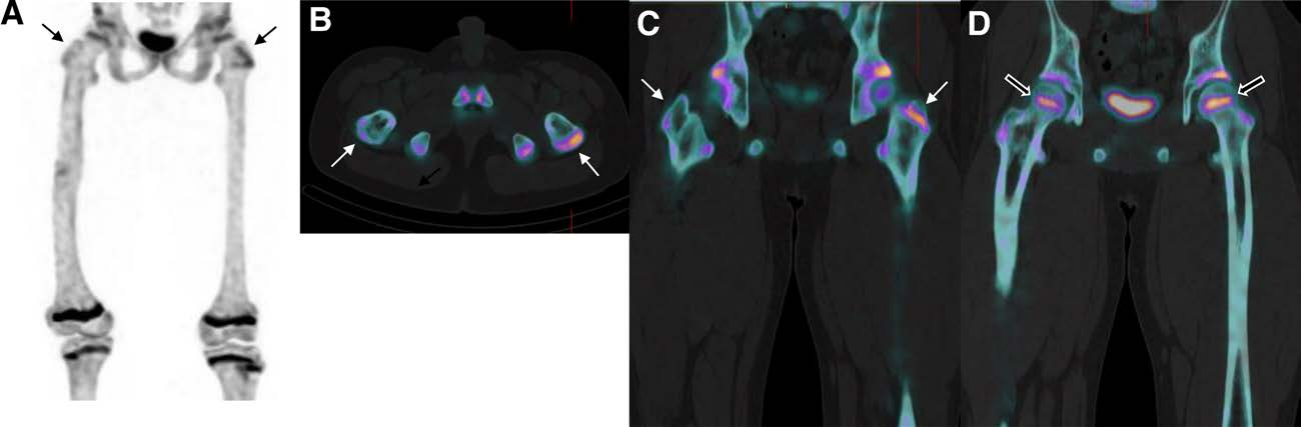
Initial bone SPECT/CT taken along with a whole-body bone scan. Radioactivities of the right greater trochanter and right femoral head were lower than those of the contralateral femur. Arrows show radioactivity in the growth plates of the greater trochanters, while empty arrows indicate those of the femoral heads. (A: maximal intensity projection (MIP) image; B and C: axial and coronal fusion images of the greater trochanters; D: coronal fusion image of the femoral heads).
